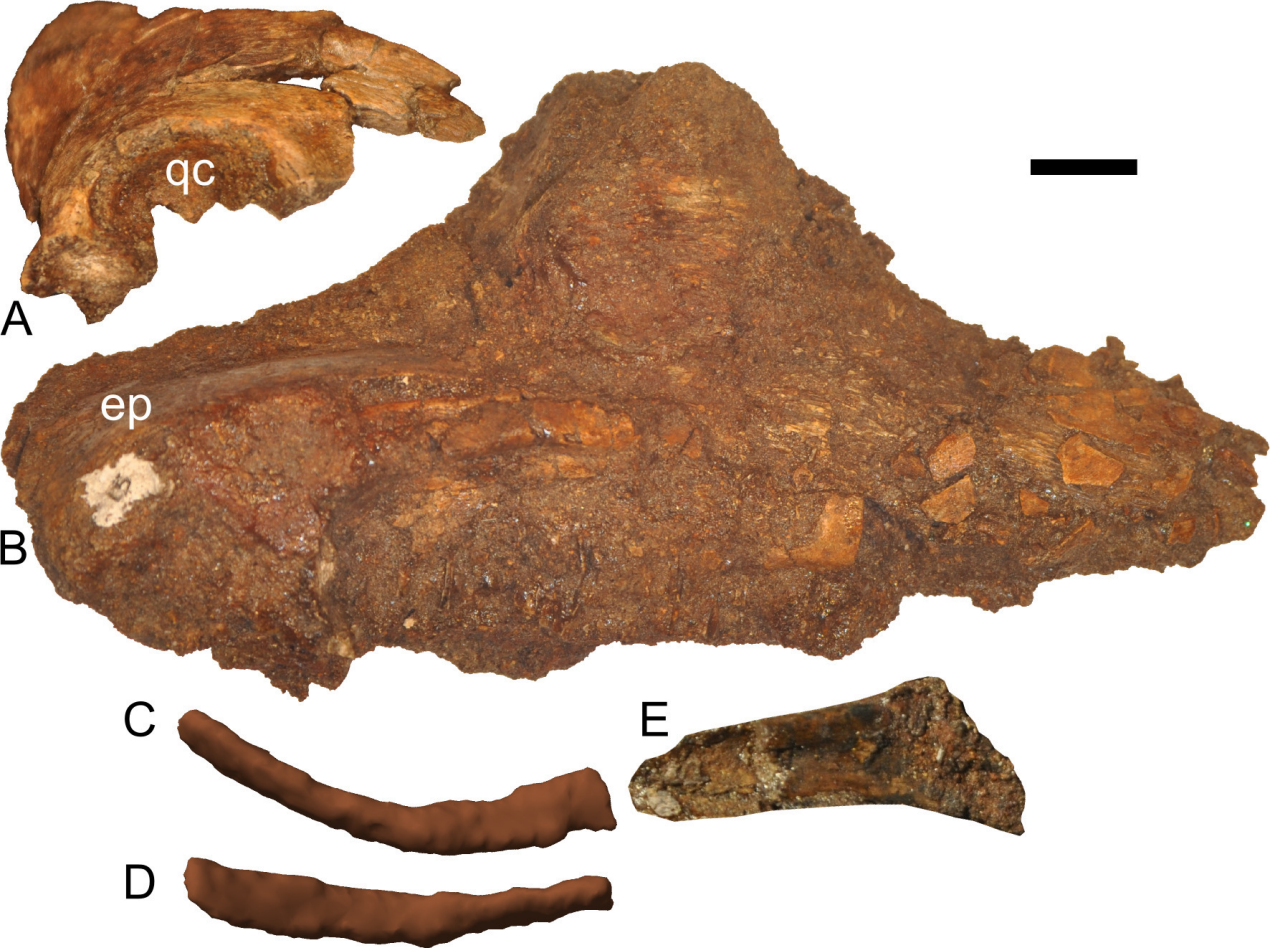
Disarticulated skull elements of *Parasaurolophus* sp., RAM 14000. (A) partial right squamosal in lateral view; (B) right maxilla in lateral view; (C–E) right first ceratobranchial in lateral (C, E) and dorsal (D) views. (C) and (D) are reconstructed from CT scan data. (C) includes the caudal portion of the element, and (E) includes the rostral portion, with the relative position of the two parts approximating their original relationship. Abbreviations: ep, ectopterygoid; qc, quadrate cotyle. Scale bar equals 1 cm.
